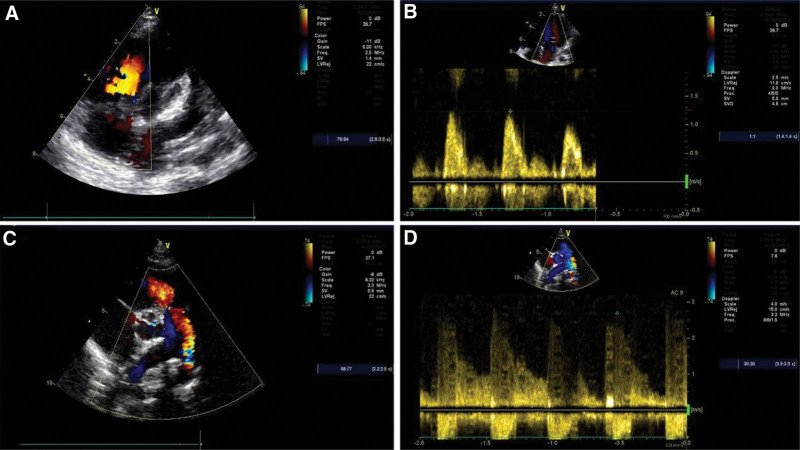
Ultrasonographic findings of the twin probands in this family line. (A and B) Proband III.9, ultrasonographic images showed an unobstructed arterial duct with a left-to-right shunt at the level of the arteries, which was diagnosed as CHD. (C and D) Proband III.10, ultrasonographic images showed an unobstructed arterial duct with a left-to-right shunt at the level of the arteries, which was diagnosed as CHD.
